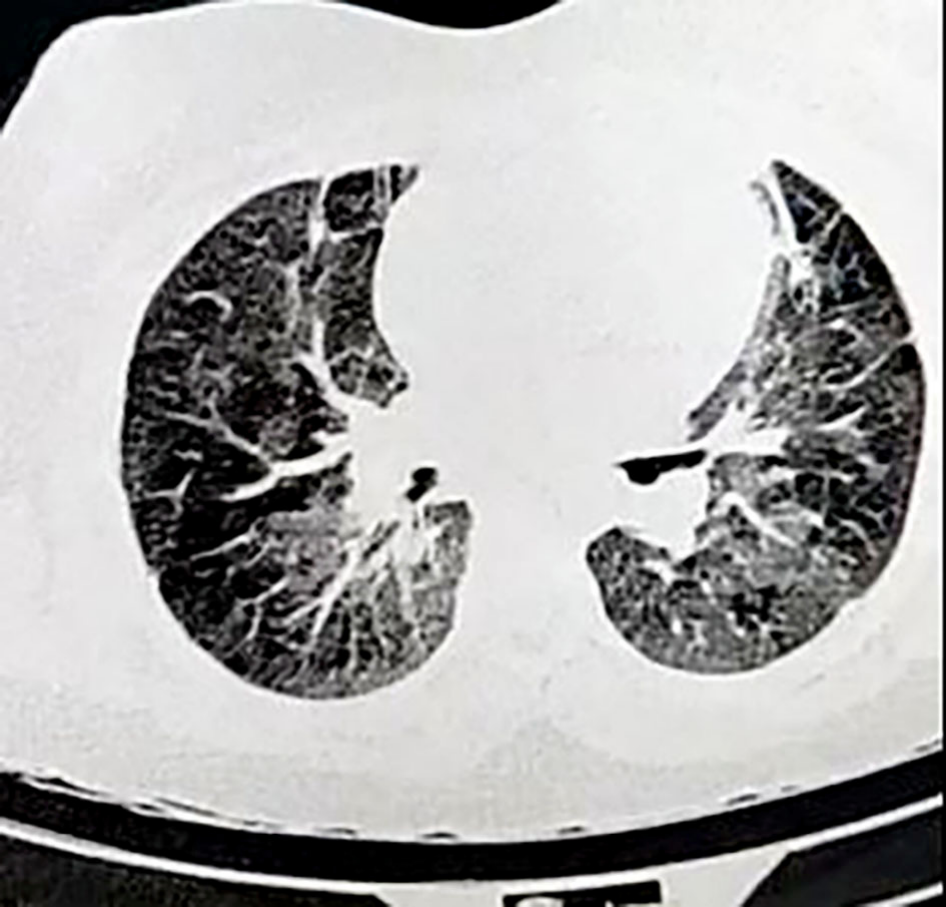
High-resolution computed tomography (HRCT) of the lungs in COVID-19 child showing bilateral multiple patchy areas of ground glass opacities.
